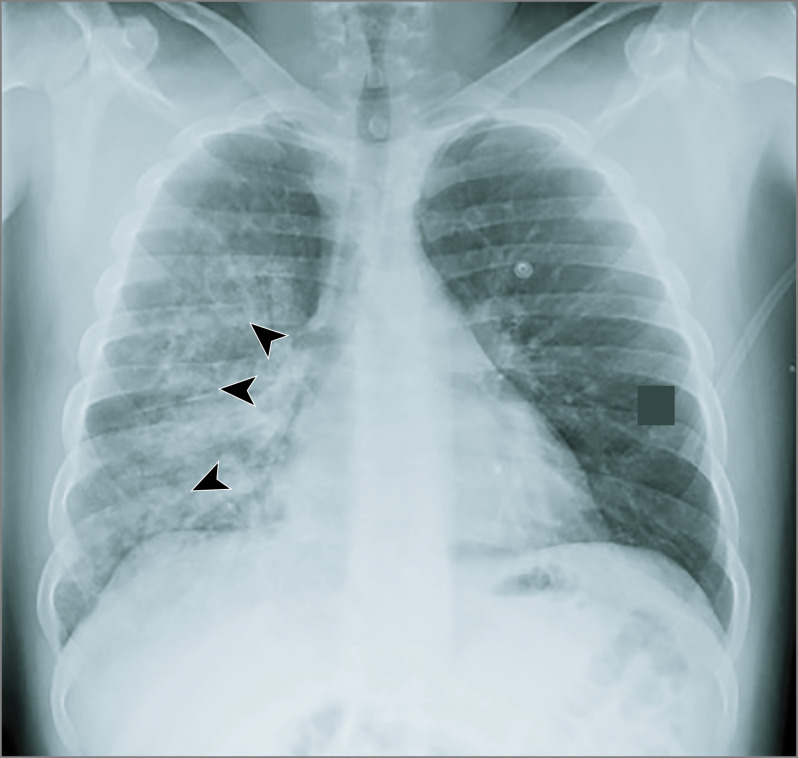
Chest Radiograph Obtained at Admission of Patient With Hypoxic Respiratory Failure and Hemoptysis Unilateral pulmonary edema (arrowheads) is noted in the clinical context of hemoptysis. Bronchoalveolar lavage findings were consistent with diffuse alveolar hemorrhage. The patient was mechanically ventilated for less than 10 hours. He left against medical advice shortly after his extubation (patient 30 in Table 2).
